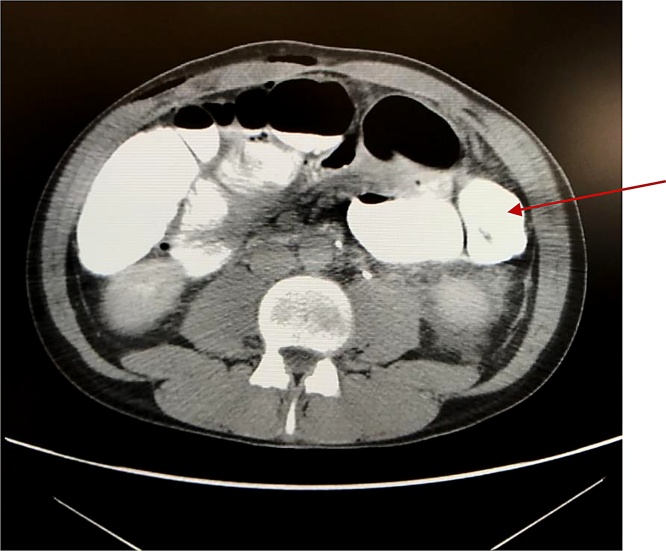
CT scan image: The arrow indicates the subsequent check-up performed after administration of gastrografin for os, showing the resolution of the obstruction.
